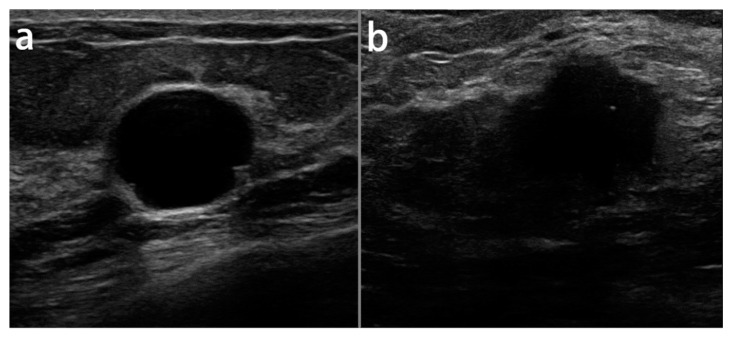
The samples of benign (a) and malignant (b) lesions in breast ultrasound images.
